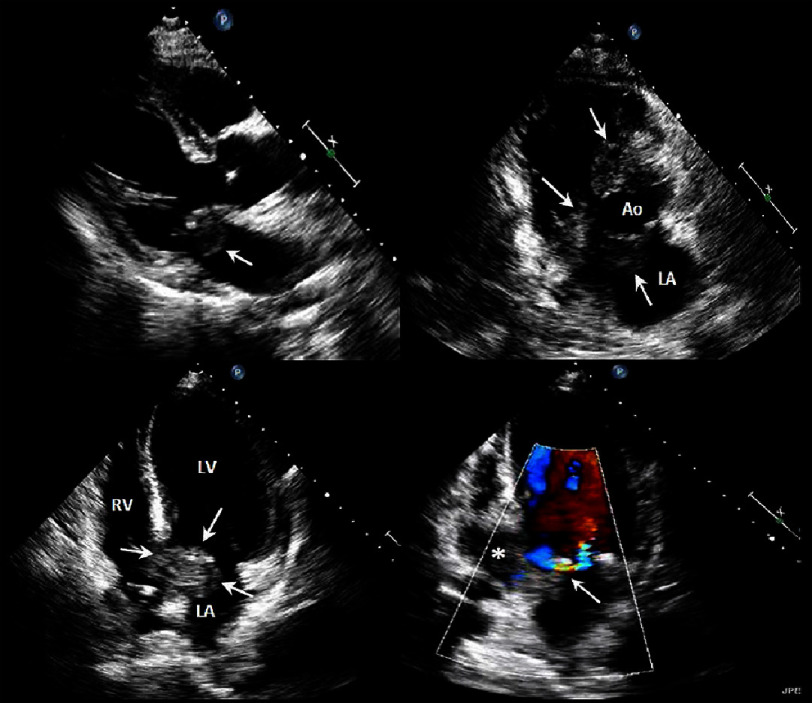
Transthoracic echocardiography. Demonstration of a mobile, invasive vegetation on the anterior leaflet of the mitral valve (Figure 1A, Video 1A, arrow). An abscess partially encircles the aorta (Figure 1B, Video 1B, arrows). The abscess involves both the interatrial and interventricular septa (Figure 1C, Video 1C, arrows). Figure 1D and Video 1D show the large abscess extending from the mitral valve annulus 4 centimeters into the inferior left ventricular wall (asterisk). Flow demonstrates a hole (Figure 1D, Video 1D, arrow) in the mitral valve leaflet.
